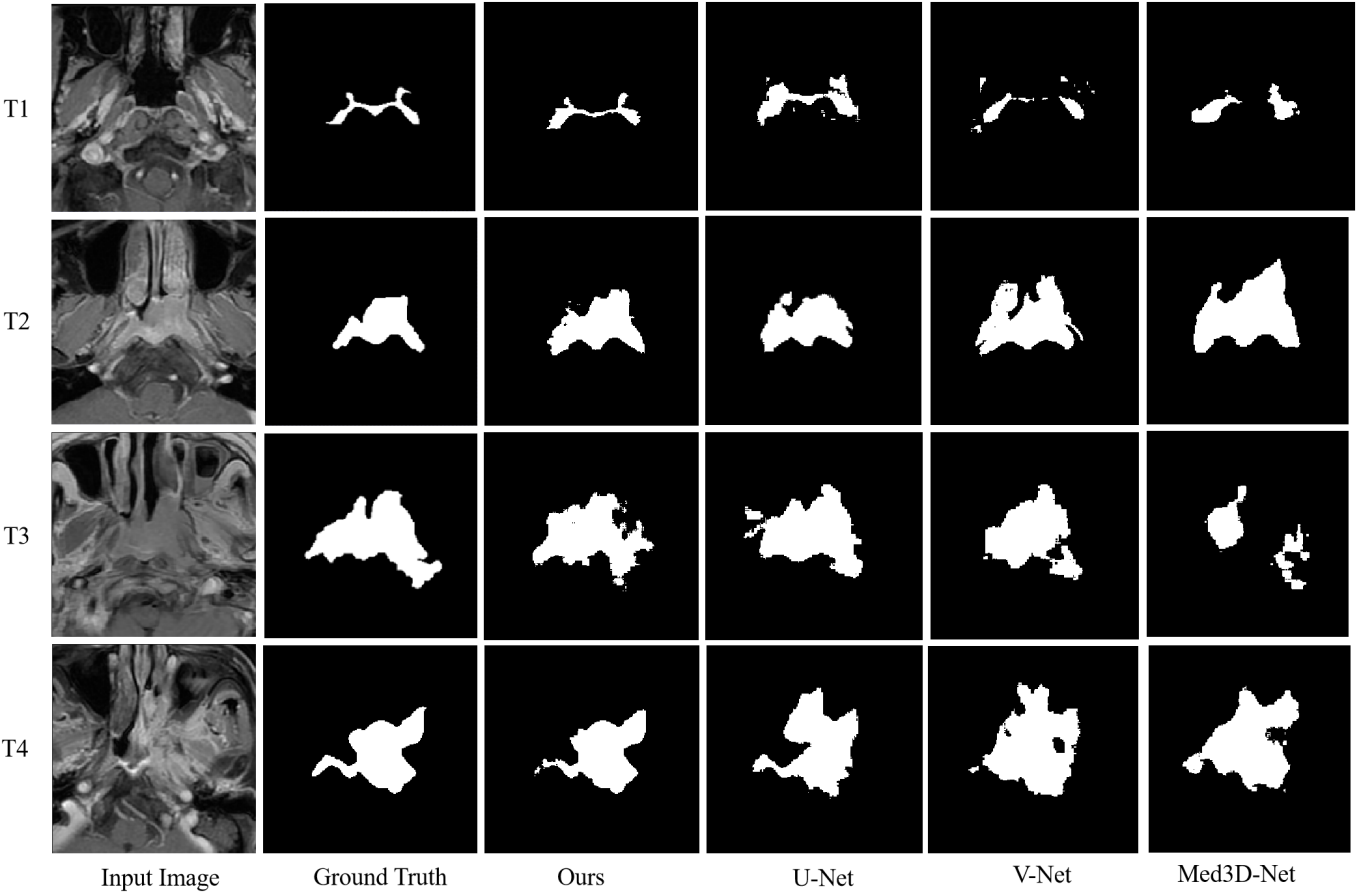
Example contrast-enhanced T1-weighted MRIs show the level of concordance for primary gross tumor volume contours between our proposed multi-task model and 3D U-Net, V-Net, and Med3D Net.
